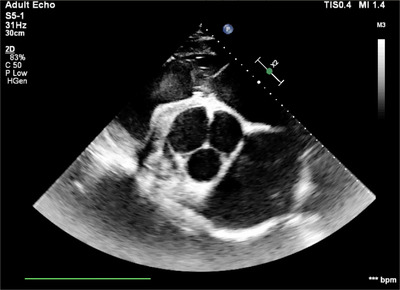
B‐mode right parasternal short‐axis left ventricular outflow tract view in diastole showing the three cusps of the aortic valve
